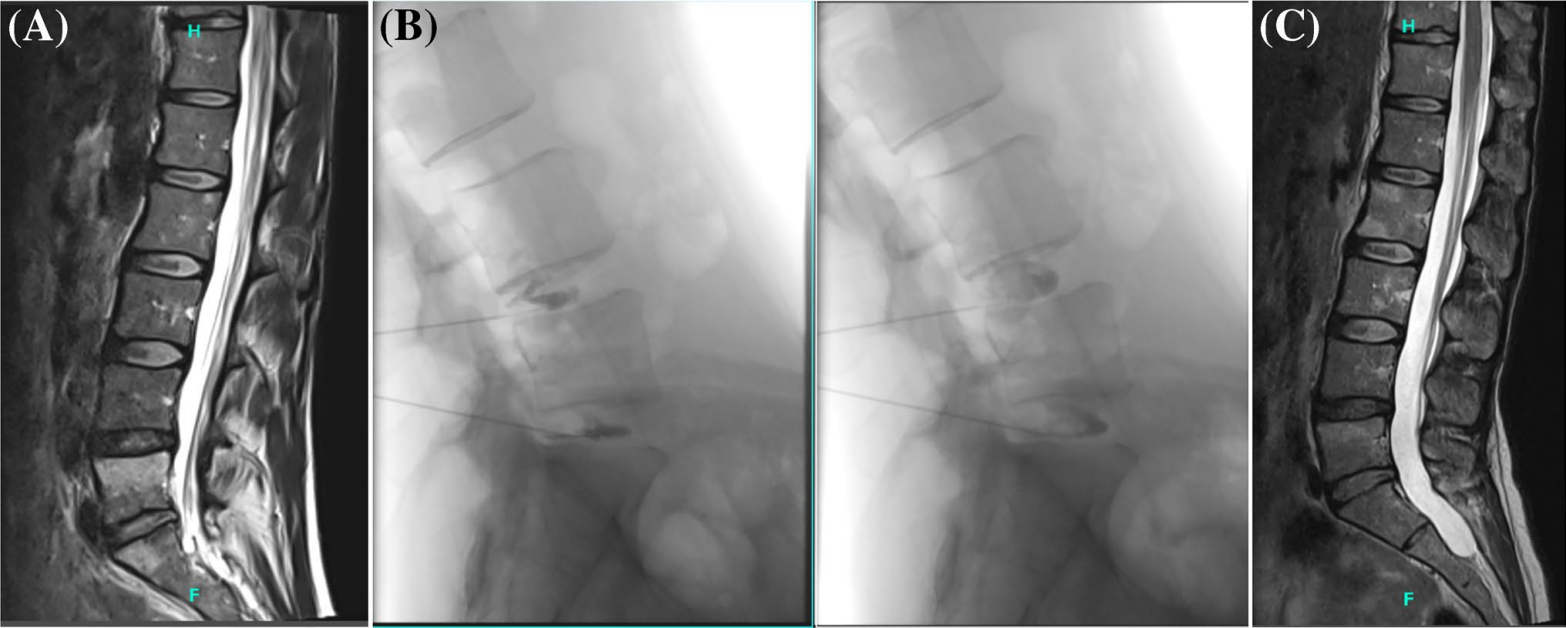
Representative magnetic resonance imaging (MRI). A MRI sagittal T2 image of Modic 1 changes at L4-5 and L5-S1 pre-treatment. B Lateral fluoroscopic images of intradiscal injection of contrast at L4-5 and L5-S1 showing annular fissures on the left and post-intradiscal leukocyte-rich platelet-rich plasma injections on the right. C MRI sagittal T2 image of improvement at L4-5 and L5-S1 at 1 year post-treatment
